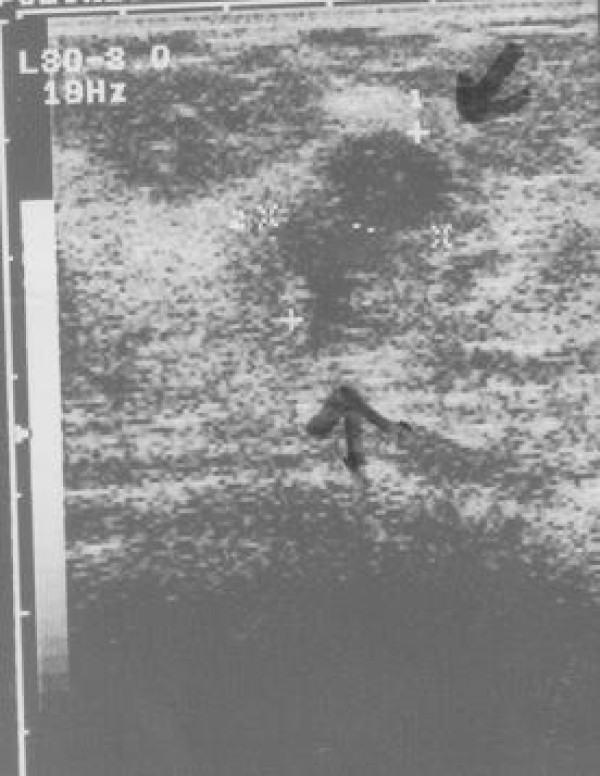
Ultrasonography. Partially well defined mass consisted of heterogenous hypoechoic foci.
